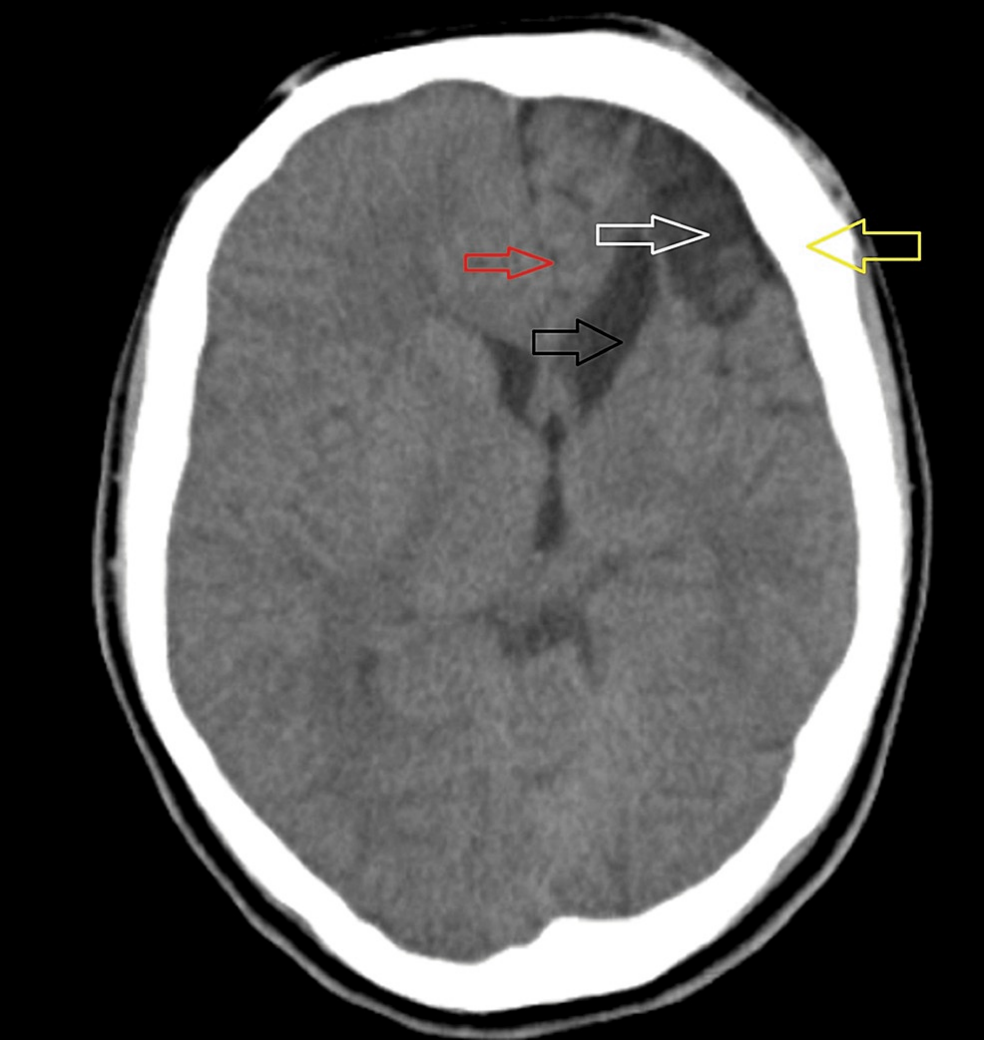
Axial non-contrast CT scan of the brain showing atrophic changes in the left cerebral hemisphere in the frontal region (white arrow), left calvarial thickening (yellow arrow), and ipsilateral lateral ventricle ex-vacuo dilatation (black arrow). Ipsilateral displacement of the falx is also seen (red arrow). CT: computed tomography
